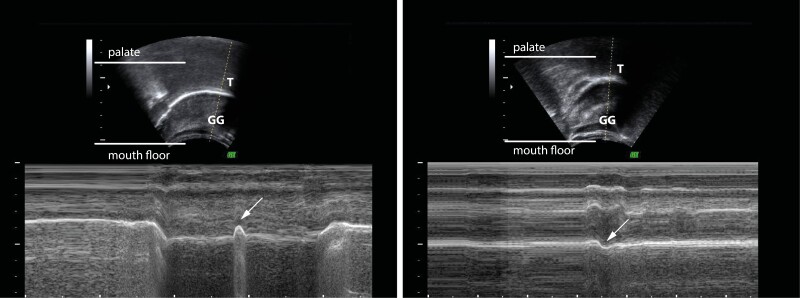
B- and M- mode tongue ultrasonogram with the ultrasound probe’s centre line set through the tip of the tongue. The white arrow shows an upward movement of the tongue tip and the genioglossus muscle in the M-mode image in Fig. 1a, which is characteristic of the somatic swallowing pattern. A downward movement of the tongue tip and the genioglossus muscle is shown by the white arrow in Fig. 1b, which is characteristic of the distinctive visceral swallowing pattern. T—tongue tip, GG—genioglossus muscle.
